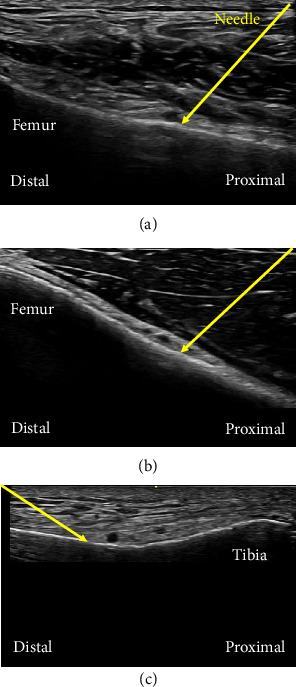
Anatomical and ultrasound images of genicular nerve blocks. Superolateral (a), superomedial (b), and inferomedial (c) genicular nerve blocks were performed. Arrows indicate needle.
